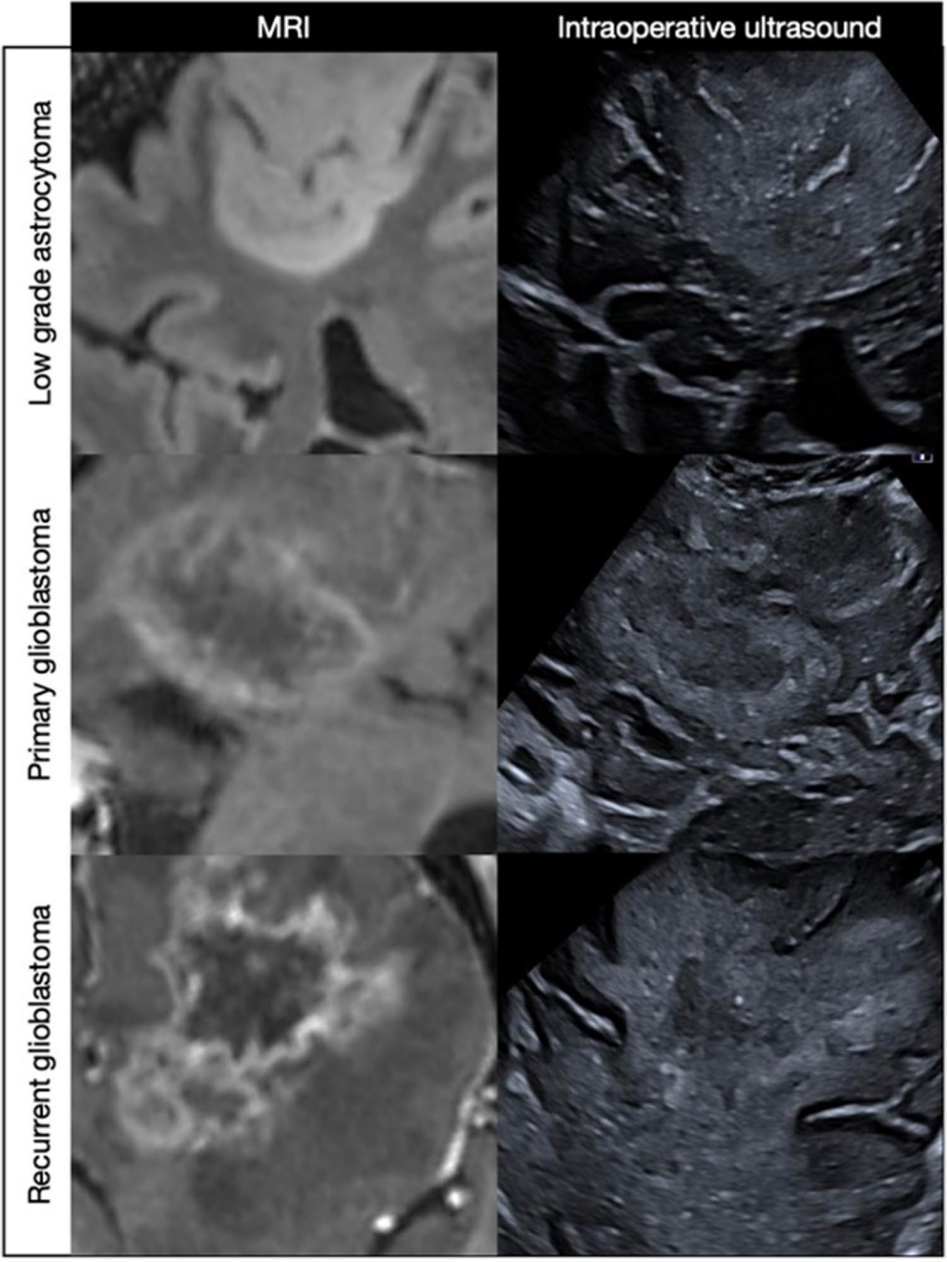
Examples of different lesions on MRI and corresponding fused ultrasound. The primary glioblastoma is the most easily visualized with clear echogenic boundaries that correlate with the enhancing rim on the preoperative post-contrast MRI. Similarly, the low-grade astrocytoma has well-defined FLAIR boundaries with corresponding matching borders on ultrasound. The recurrent glioblastoma is well defined on the post-contrast MRI but poorly visualized on ultrasound
